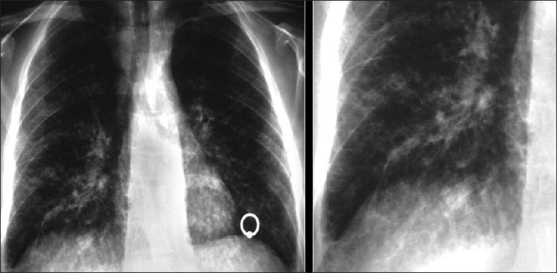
Kaposi’s sarcoma is the most common acquired immunodeficiency syndrome-related malignancy. The chest X-ray shows bilateral perihilar/lower zone reticulonodular infiltrates
